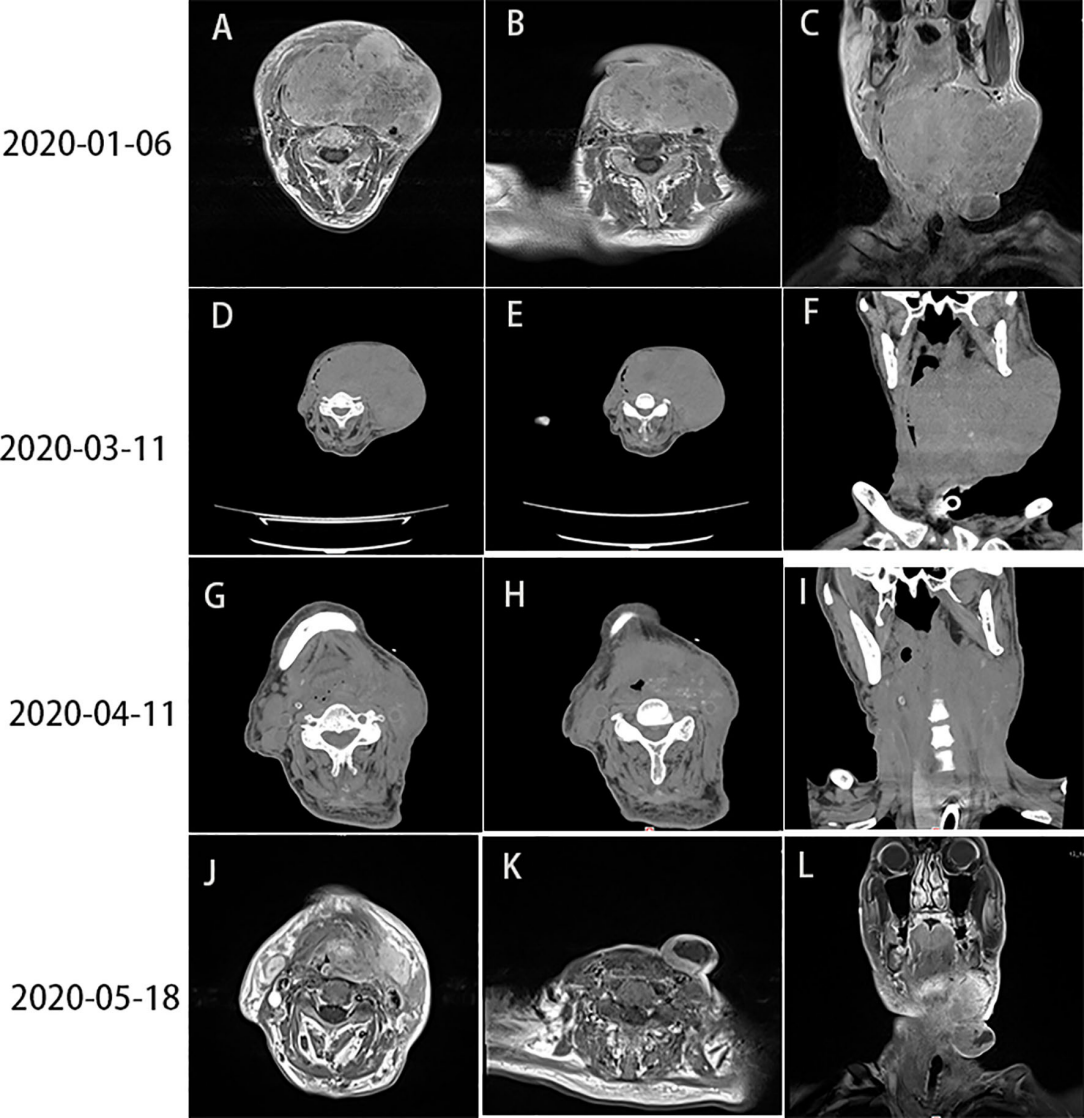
(A–C) Head and neck CT showed lymph node enlargement (10 × 12 cm) on the left side of the neck (January 6, 2020). (D–F) Head and neck CT, performed 10 days after the first cycle of treatment, showed progression of the tumor mass (13 × 10 cm) (March 11, 2020). (G–I) PR was detected after the first course of combination therapy with sintilimab and nimotuzumab (April 11, 2020). (J–L) Head and neck MRI revealed PR (May 18, 2020). CT, computed tomography; MRI, magnetic resonance imaging; PR, partial response.
